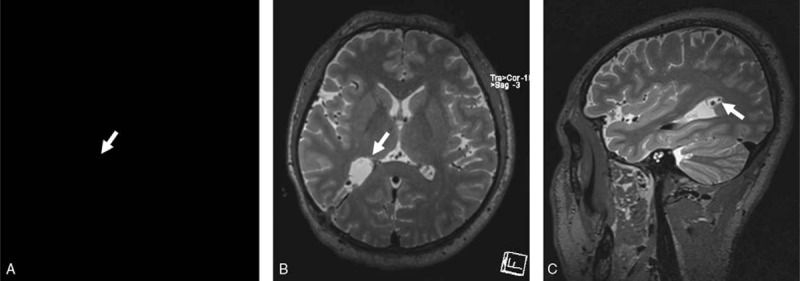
Postoperative magnetic resonance imaging showing no residual tumor (white arrows).
